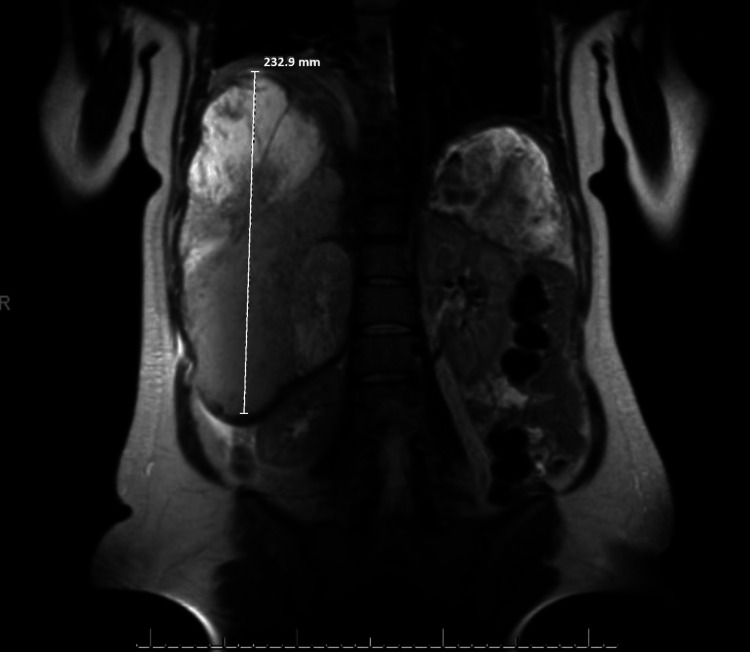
Coronal MRI of the abdomen and pelvis showing 23.3 cm (232.9 mm) cystic liver mass MRI: magnetic resonance imaging
